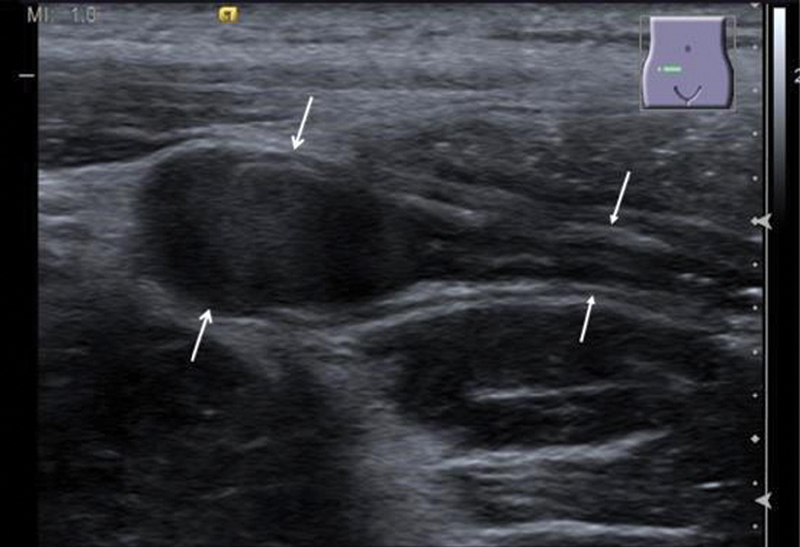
There is marked thickening of parts of the appendix up to a diameter of 7 mm (open arrowheads) compared with the normal adjacent part of the appendix (closed arrowheads). Technique: B mode sonography and color-coded sonography, Siemens ACUSON S2000, 14 MHz linear probe.
